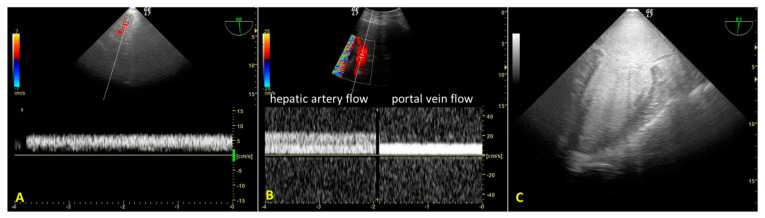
(A). Transesophageal color Doppler echocardiography with transgastric approach during the initial phase of regional extracorporeal support. Slow hepatic artery flow is sampled due to incomplete aortic occlusion (continuous right hepatic artery flow velocity = 7 cm/s). Portal vein flow was absent. (B). Color Doppler ultrasound with convex probe after complete aortic occlusion. Right hepatic artery flow was increased (velocity 25 cm/s) and the portal flow was sampled (velocity 12 cm/s). (C). Absence of flow in the left ventricle and atrium after complete aortic occlusion (mid-esophageal two-chamber view).
